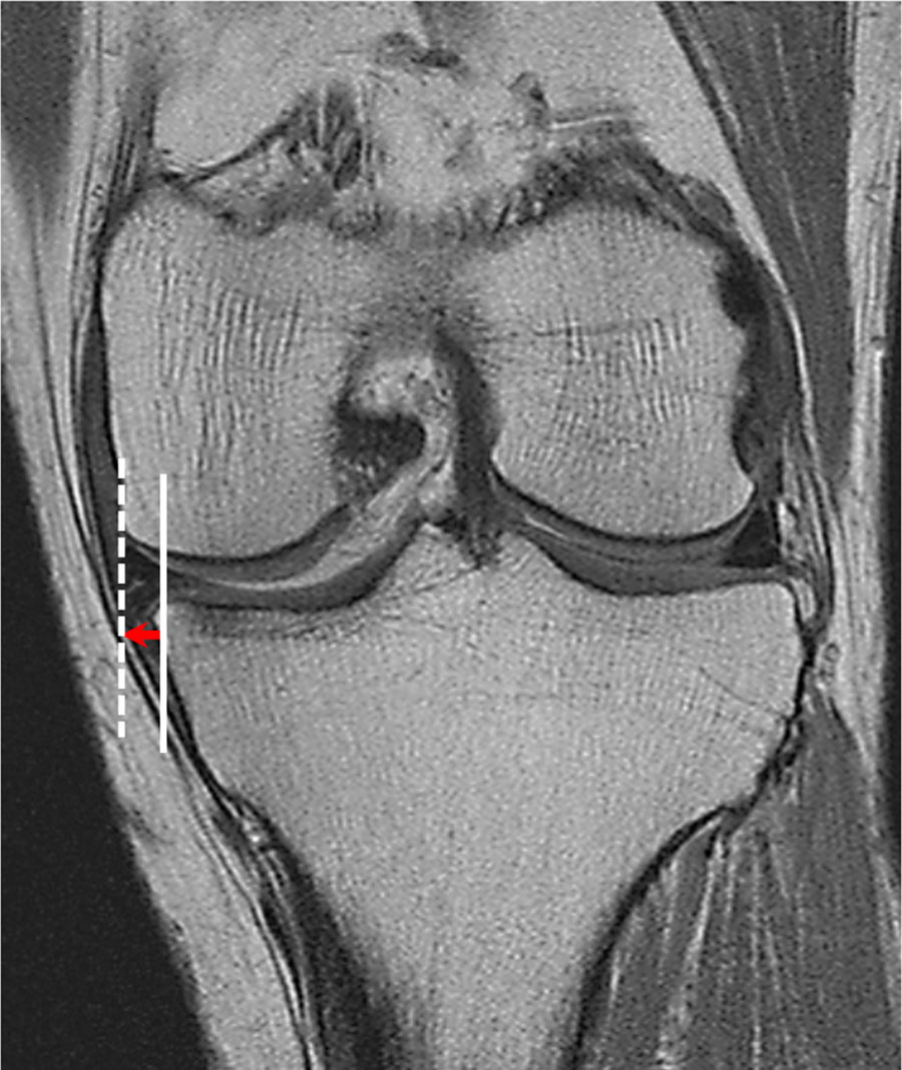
Measurement of MME. MME is measured using an MRI coronal slice where the highest medial tibial eminence is observed. MME is defined as the distance from the medial margin of the tibia (white line), excluding osteophytes, to the medial margin of the medial meniscus (white dashed line). MME, medial meniscus extrusion; MRI, magnetic resonance imaging.
